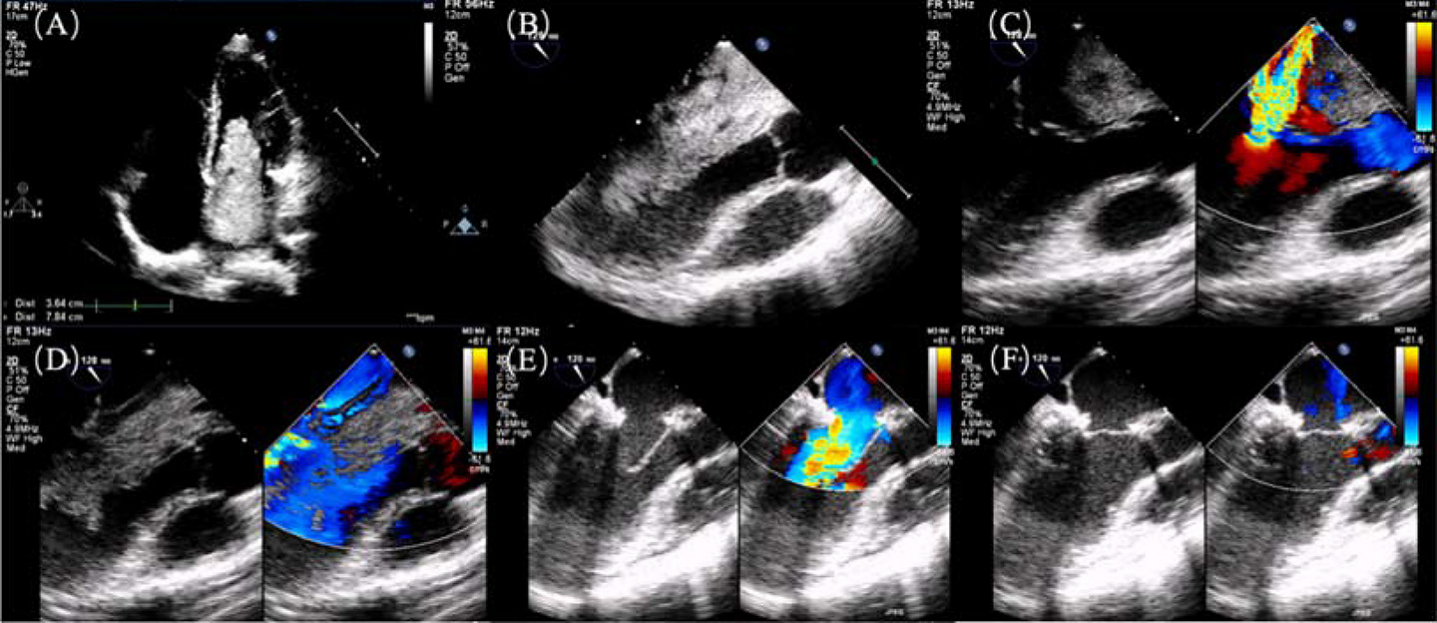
(A) Preoperative echocardiography showed the size of the left atrial myxoma was 92 × 43 mm, which was the occlusion of the mitral valve orifice. (B) ME LAX view showed that the left atrial myxoma occupied almost the entire left ventricular inflow tract during diastole and mitral valve. (C) ME LAX view showed left atrial myxoma pushed into the left atrium as a whole during systole and with severe mitral regurgitation. (D) ME LAX view showed the atrial myxoma was obstructing the left ventricular inflow tract, causing inflow tract obstruction during diastole. (E) After mitral valve annuloplasty, the mitral valve opened well during diastole. (F) There was no mitral regurgitation after mitral valve annuloplasty.
